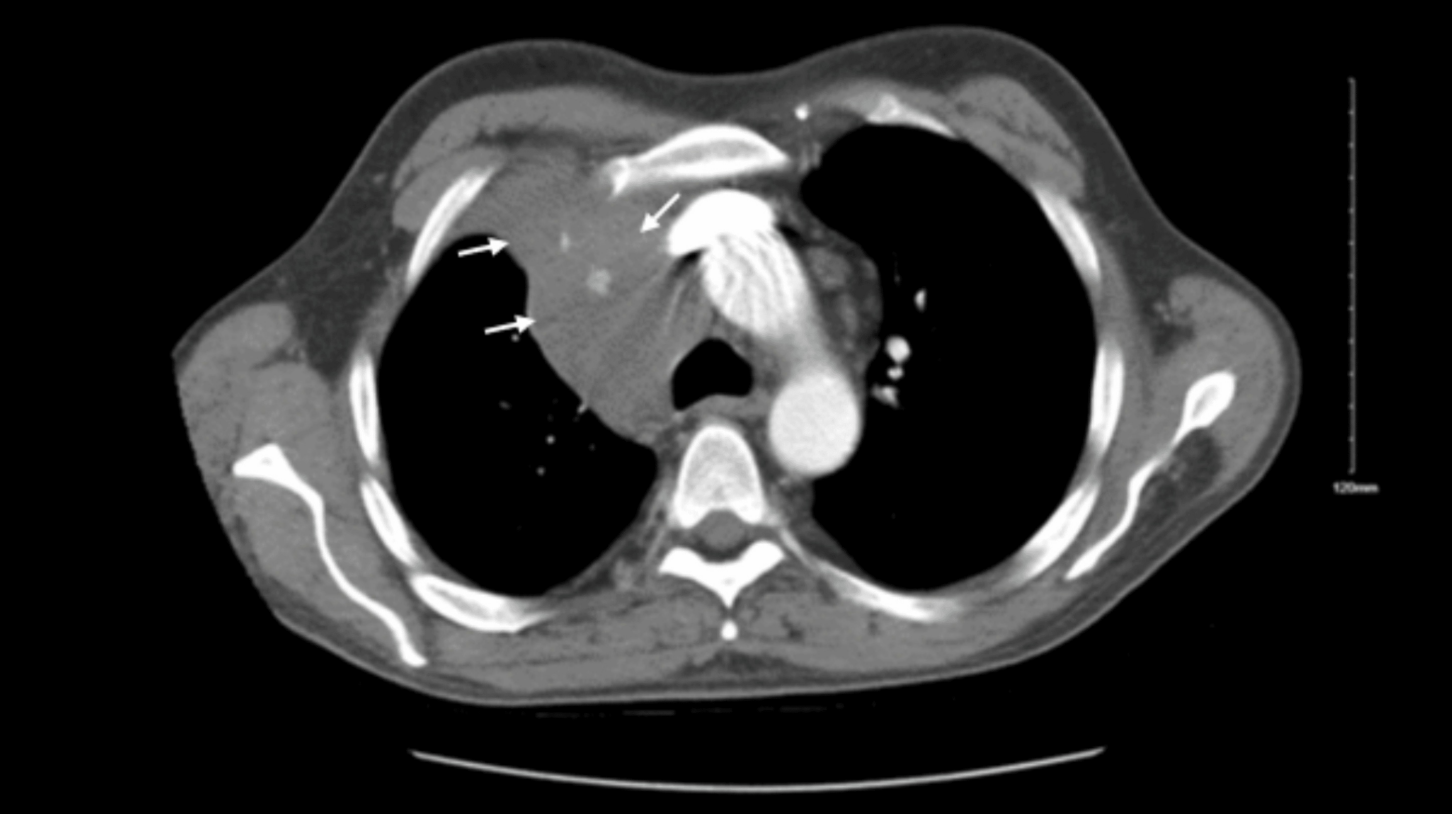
CT scan of the chest, six months post-treatment, showing a reduction in the size of the lymphoma. CT: computed tomography.
